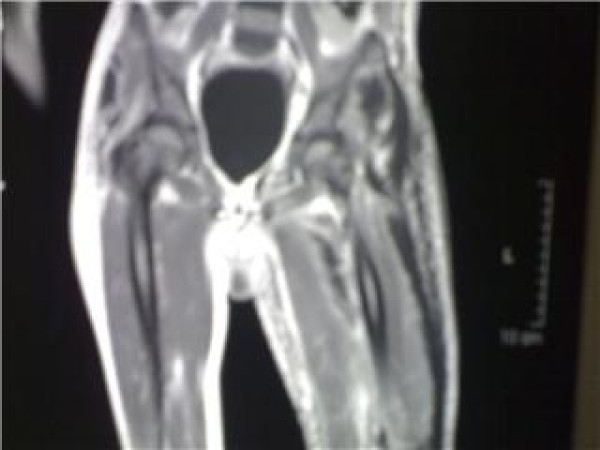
MRI of the left thigh revealed extension of inflammation along the fascial plains.
